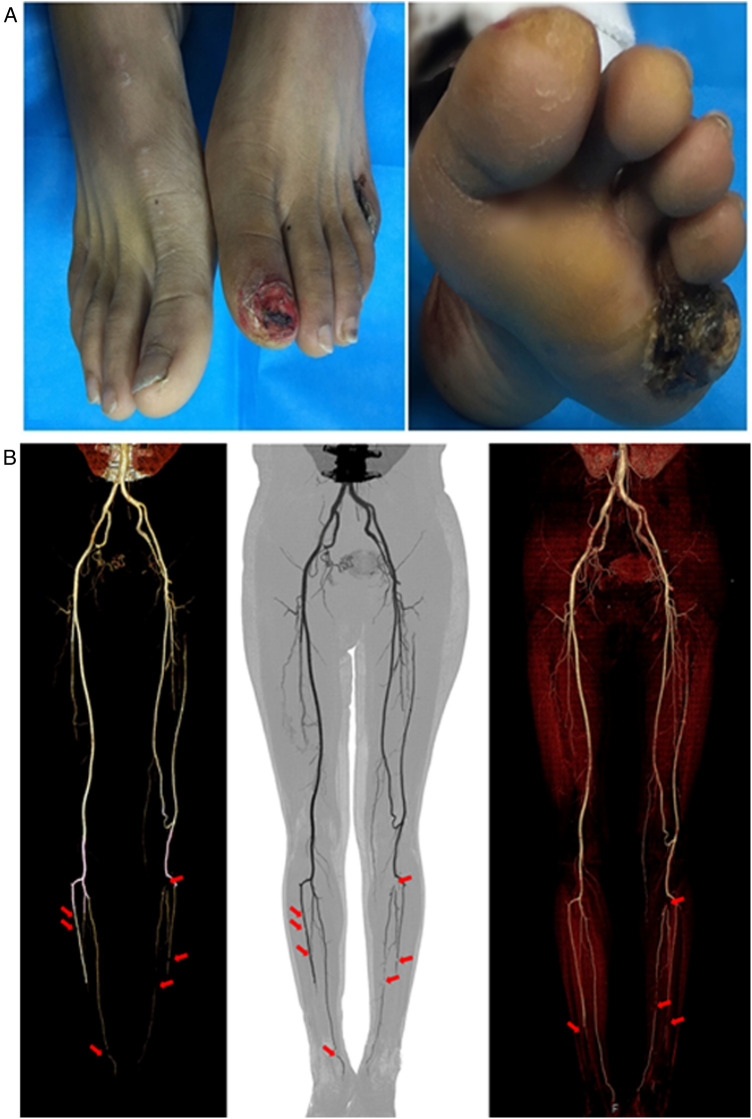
(A) A photograph of the patient before treatment, showing his lower limb chronic ischemic ulcer wound. (B) Computed tomographic arteriography (CTA) shows vasculitis of large and medium arteries noninvasively (red arrows).
